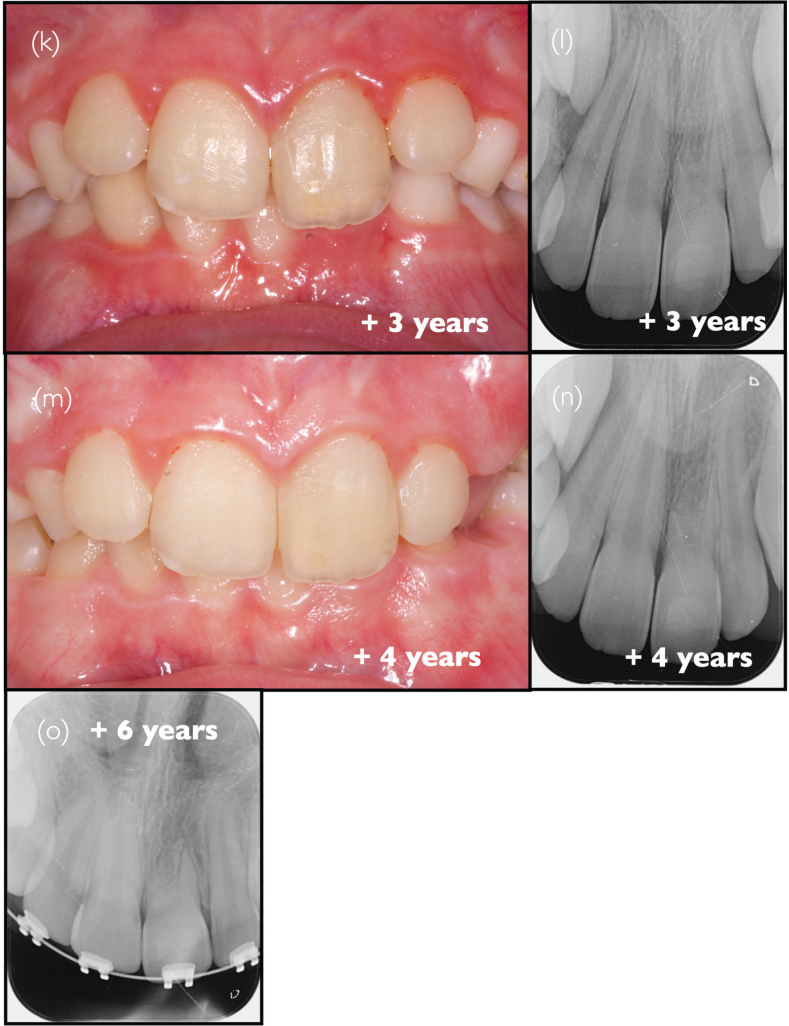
Clinical case #2 (A, B) Pre-operative intraoral photograph and radiograph. (C) Intraoperative radiograph showing the application of a calcium hydroxide agent. (D) Intraoral photograph taken 1 month after the initial visit. (E) Radiograph following the completion of the revitalisation procedure. (F–O) Post-operative follow-up intraoral photographs and radiographs taken periodically from 3 months to 6 years after the procedure.
